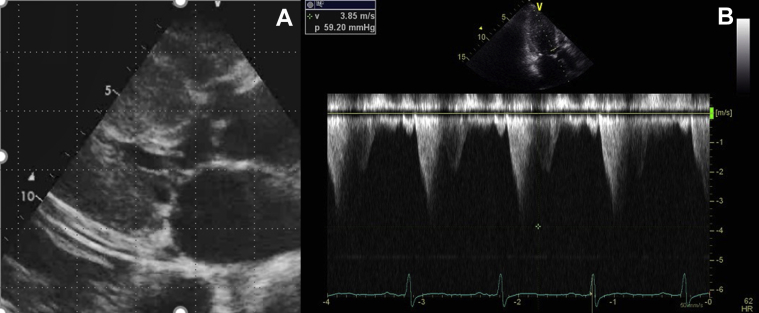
Transthoracic Echocardiography Findings Almost 1 Month Before Admission (A) Left ventricular (LV) ejection fraction is approximately 70%, and diffuse severe LV hypertrophy excluding the basal posterior wall is observed. The maximum LV wall thickness was 20 mm. (B) Systolic anterior motion of the mitral valve is also observed, and the accelerated blood flow of the LV outflow tract was approximately 3.85 m/s.
